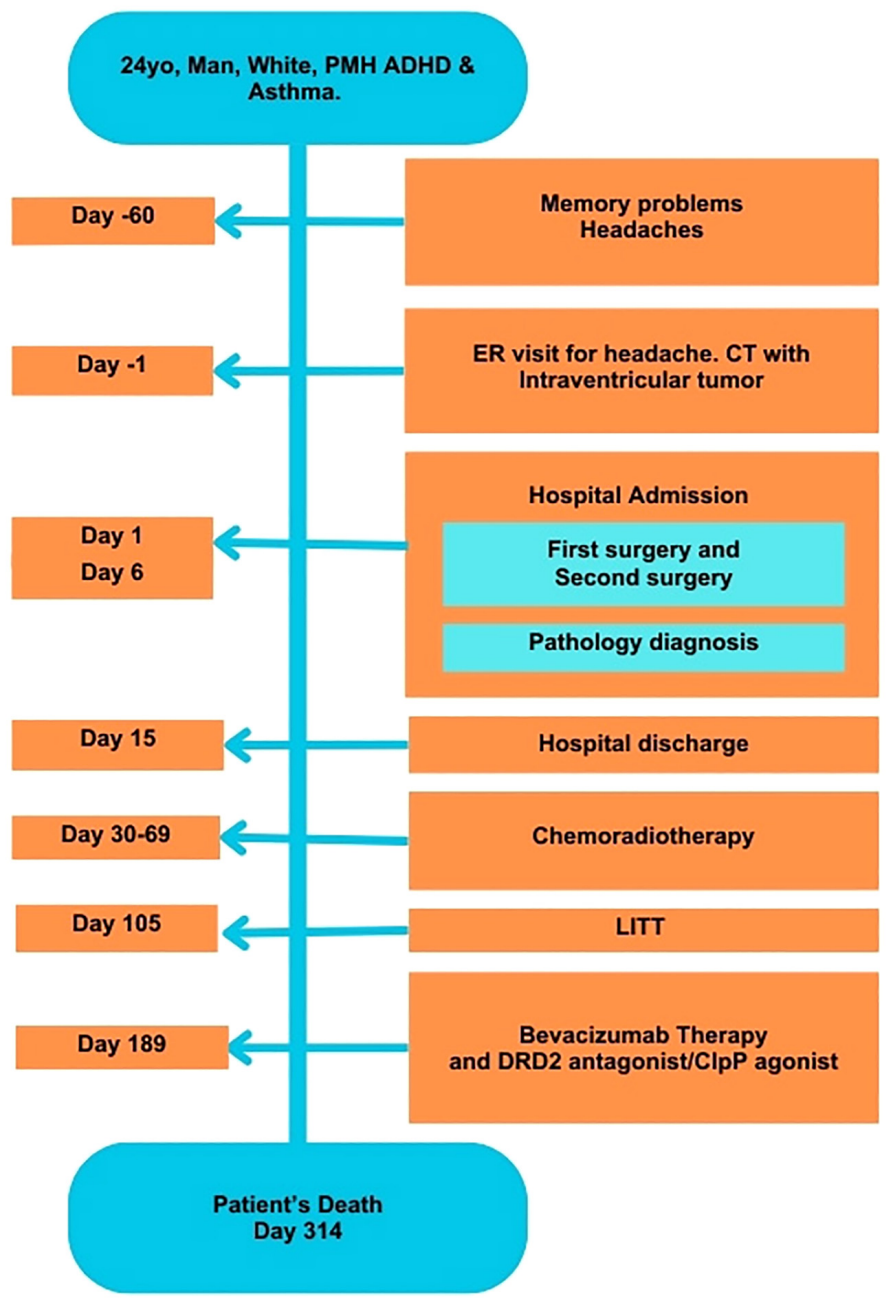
Timeline of development of symptoms and tumor treatments. ADHD, Attention Deficit Hyperactivity Disorder; ER, Emergency Room; CT, Computed tomography; LITT, Laser Interstitial Thermal ablation; PMH, Past Medical History.
